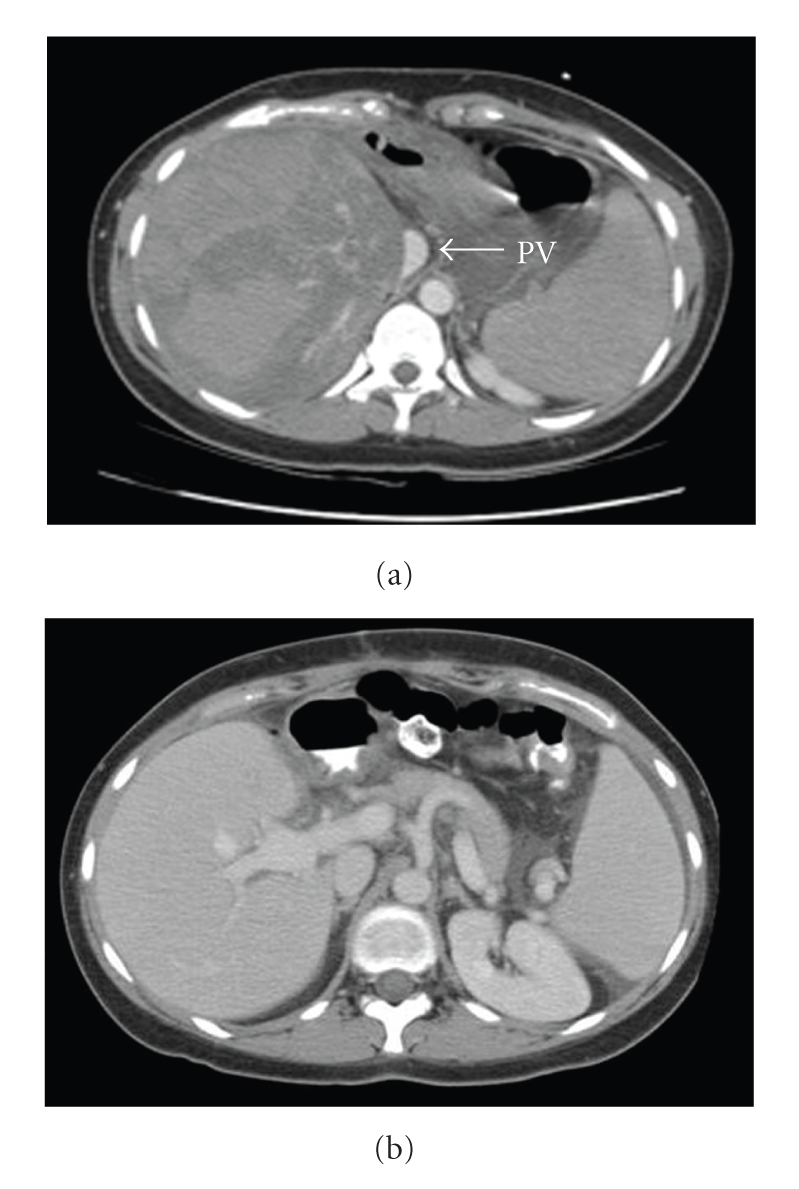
(a) CT scan demonstrating extensive intraparenchymal hematoma and compression of portal vein (PV) branches; (b) for comparison, a CT scan from the same patient from 2 years prior shows the normal appearance of a right lobe graft with normal portal vein branches.
